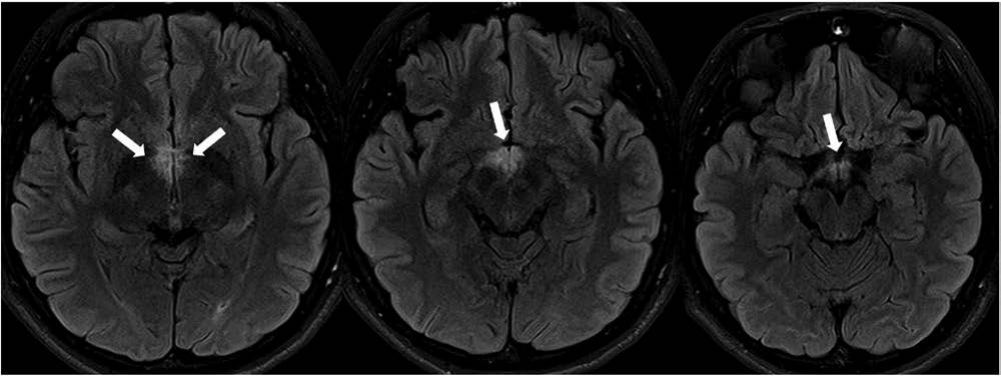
Brain magnetic resonance imaging (MRI) on the 3^rd^ d of admission. Fluid-attenuated inversion recovery imaging (FLAIR) showed symmetrical hyperintensities in the anteroinferomedial thalamus, hypothalamus, mamillary body.
